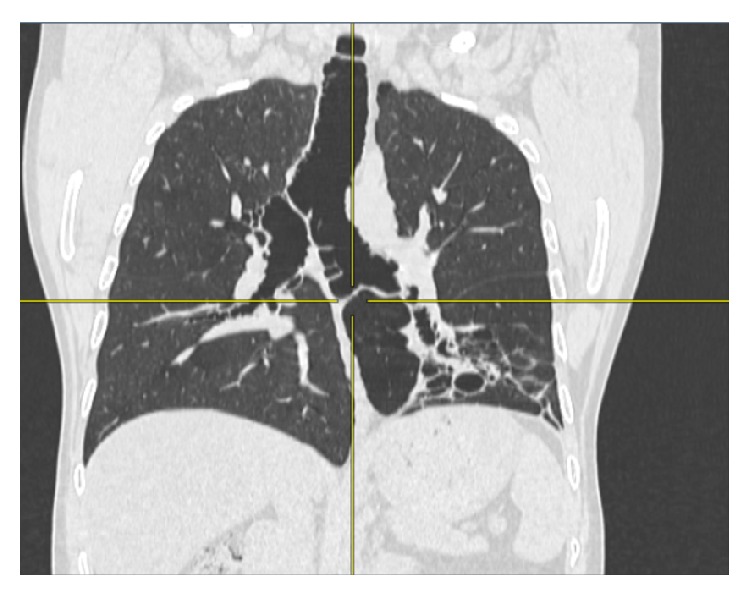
Transverse section of CT showing the grossly dilated trachea and main bronchi.
